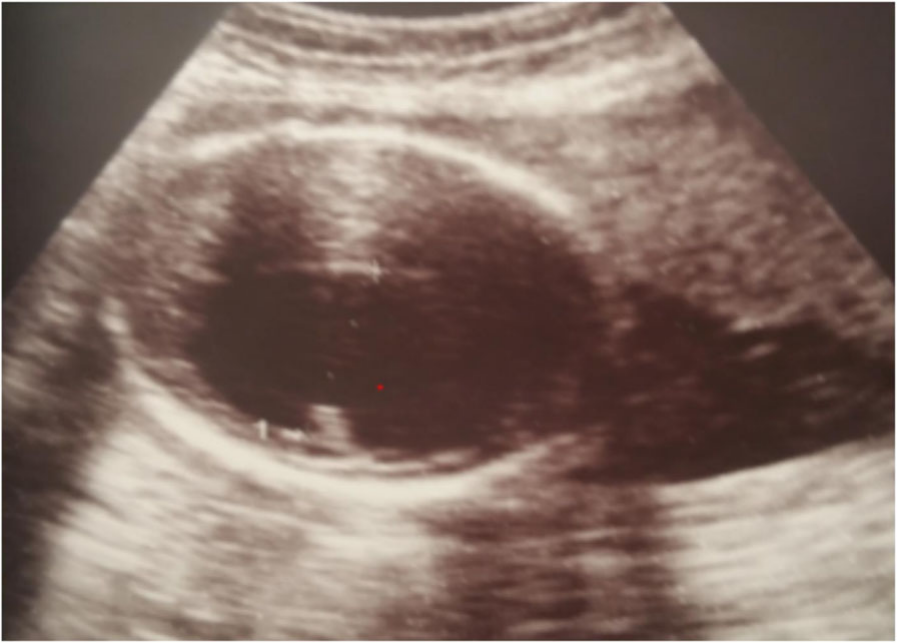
Ultrasound image shows axial view of semilobar holoprosencephaly (see partially formed falx cerebri and absent cavum septi pellucidi)
